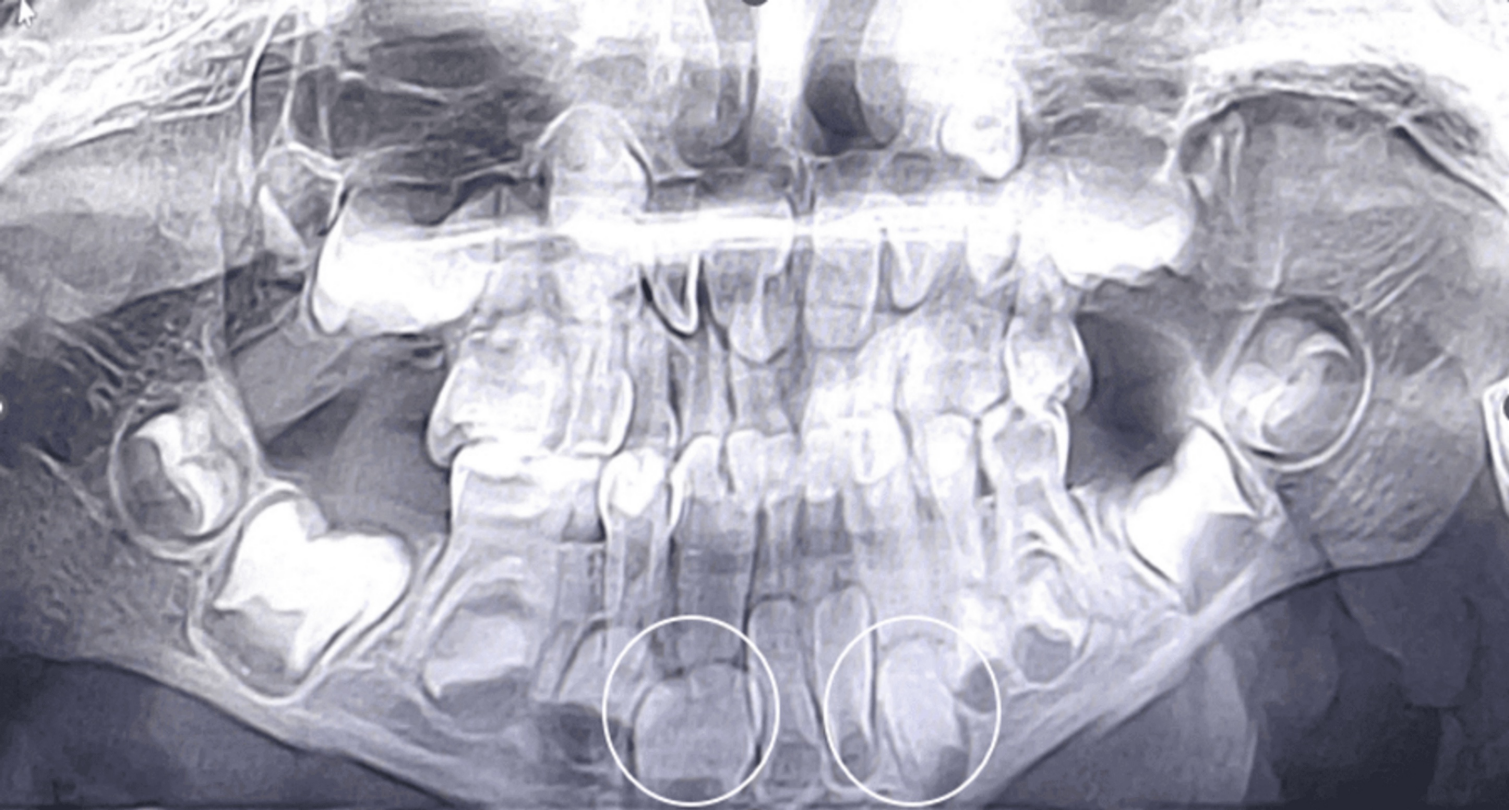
Case 2 radiographic image Orthopantomogram revealing fusion with respect to 72,73 and 82,83; Agenesis of tooth buds 32 and 42
